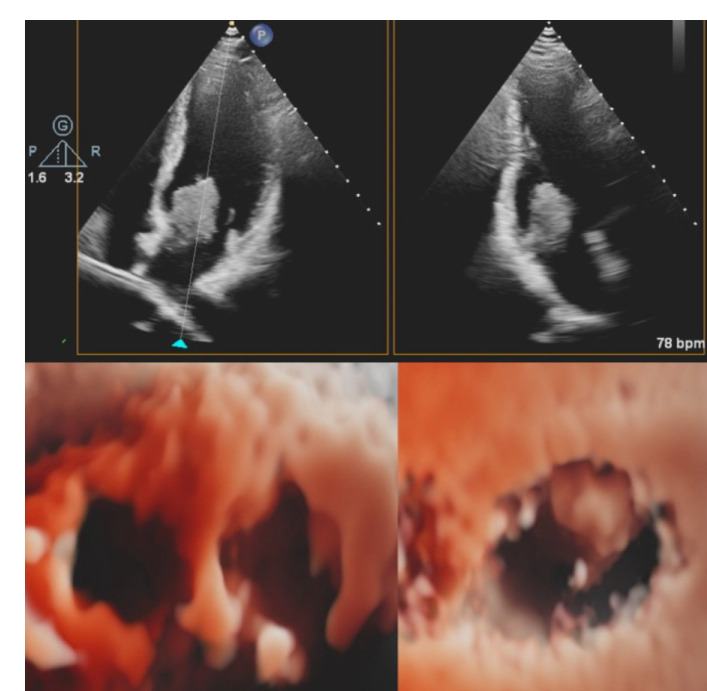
2D transthorachic echocardiography images and 3D reconstruction of the left atrial mass.
